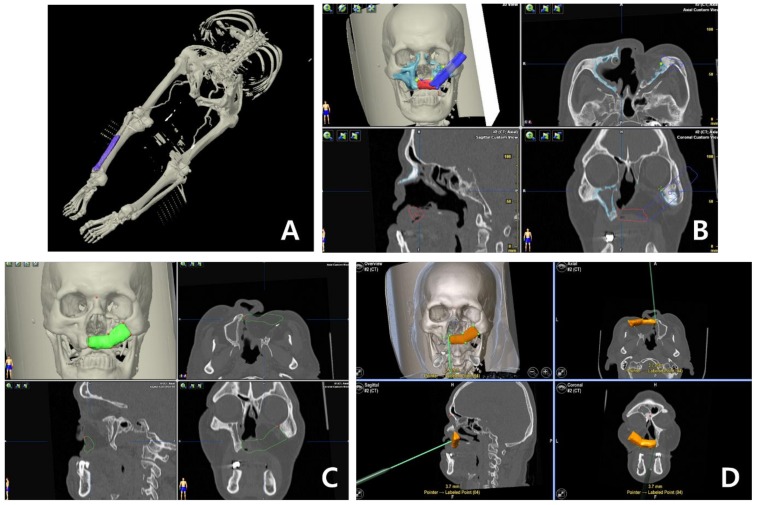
Preoperative procedure involving computer-aided design/computer-aided manufacturing (CAD/CAM) system with navigation system (BrainLab^®^) in order to enhance the accuracy and to reduce the operation time: (A) Lower extremity angio CT, (B) image export to the CAD program (BrainLab^®^), (C) the fibular bone is adjusted to the mirror image of contralateral side, and (D) navigation guide for the intraoperative procedure.
